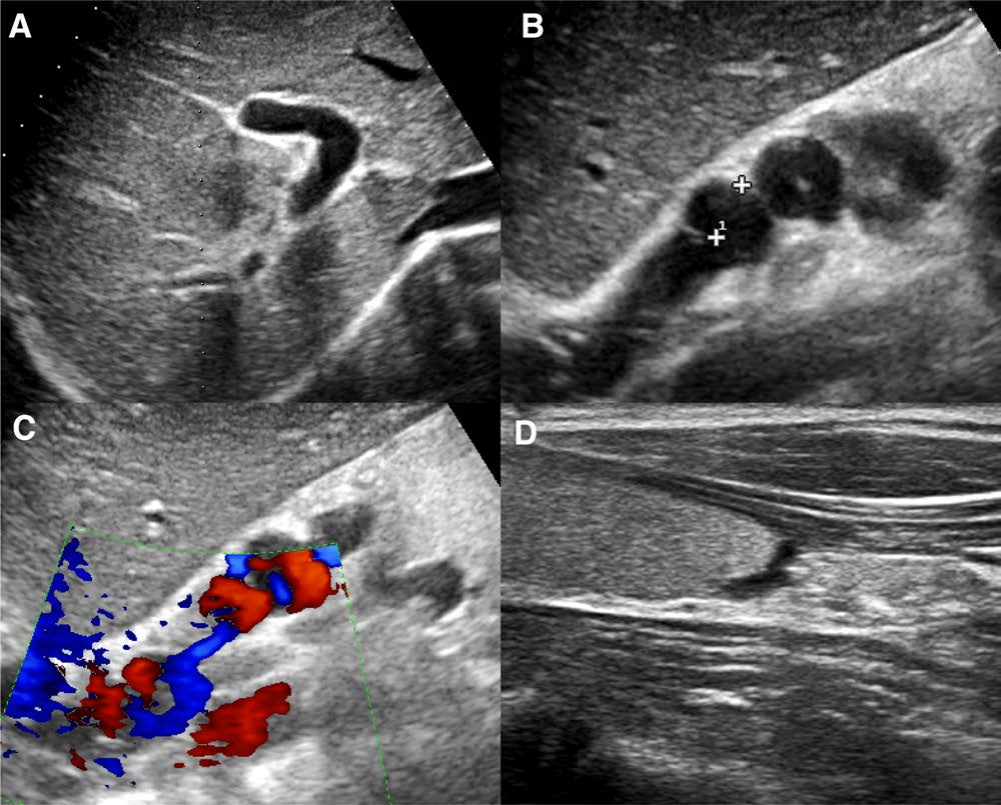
Ultrasound images. (A) Routine ultrasound showed hyperechoic and heterogeneous echogenicity of the liver, with no apparent dilation of the bile ducts. (B) Gastric filling ultrasonography showed a twisted and dilated tubular structure in the lower esophagus to the gastric fundus. (C) Color Doppler Flow Imaging of gastric filling ultrasonography showed twisted and dilated tubular structure filled with blood flow signals. (D) High-frequency probe indicated no apparent esophageal varices behind the thyroid in the cervical segment.
